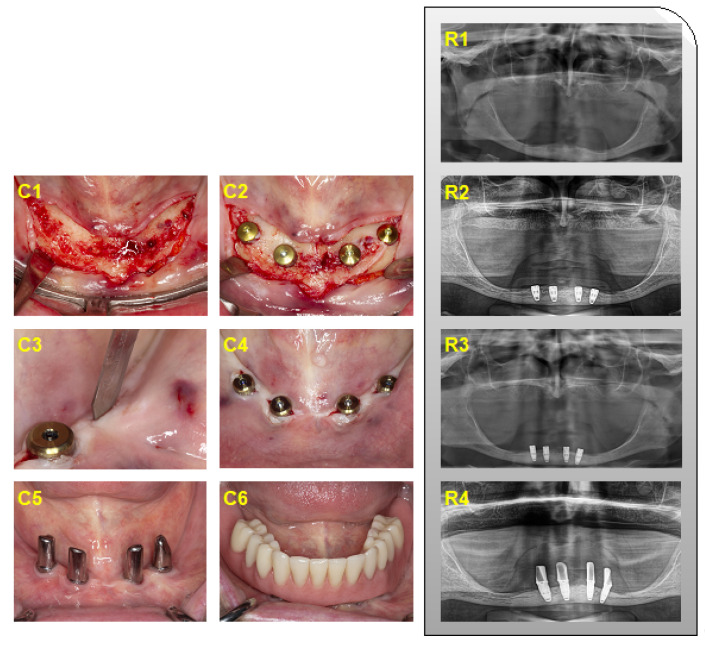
Clinical (C1–C6) and radiographic (R1–R4) presentation of interforaminal implant placement in pristine bone. (C1) Intraoperative situs, (C2) implants connected to cover screws, (C3) uncovering of implants, (C4) implants connected to healing abutments, (C5,C6) at the time of prosthetic reconstruction; (C5) implants connected to telescopic crowns, and (C6) final prosthetic outcome. (R1) Preoperative situation, (R2) after implant placement, (R3) after uncovering of the implants, and (R4) implant loading for 1.5 years.
